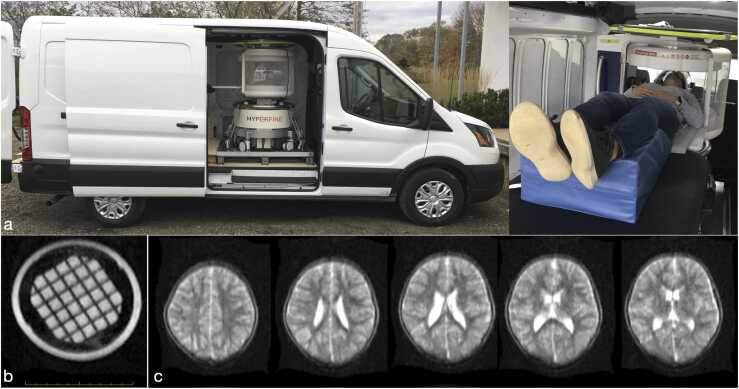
Mobile low field MRI using the 64mT Hyperfine system installed in a customized cargo van (a). Despite its low field and portable nature, the device provides sound imaging abilities without geometric distortions (b) and with image quality and contrast nearing 1.5 T quality (c).
